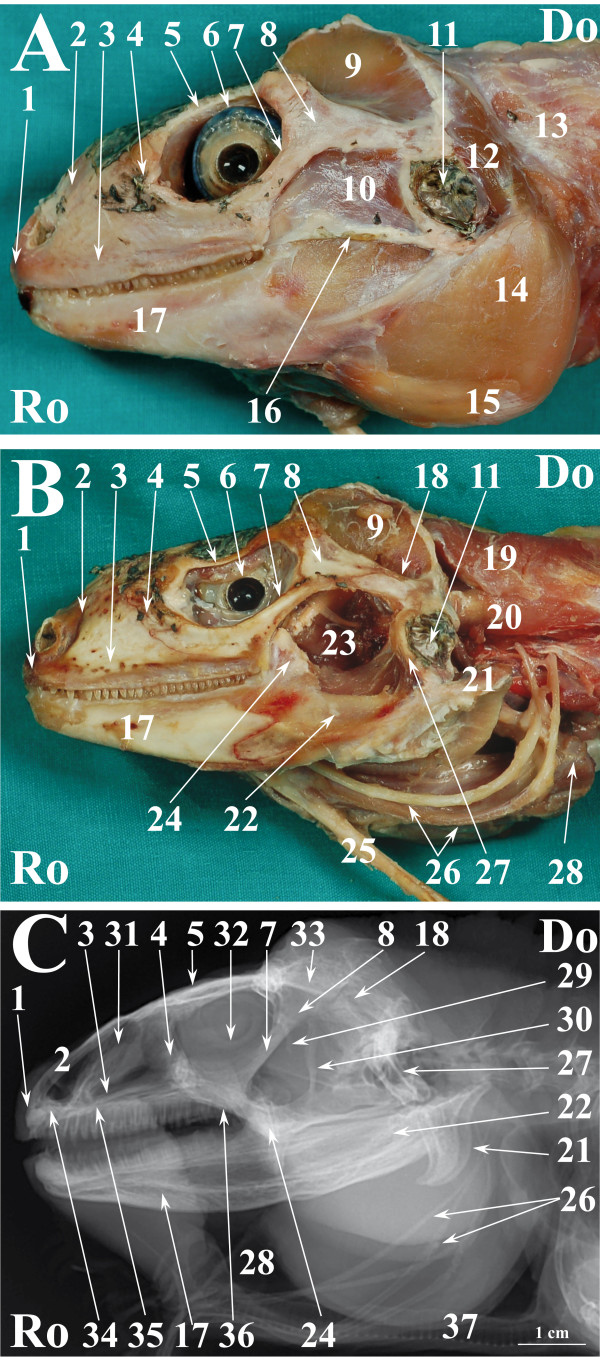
Normal radiographic anatomy of the head of the green iguana in LL projection.A. LL photographic image in a superficial plane of the stratigraphic dissection of the head of an iguana (only skin was removed). B. LL photographic image in a deep plane of the stratigraphic dissection of the head of an iguana. C. LL radiographic projection of the head of an iguana. Ro is rostral, Do is dorsal. 1. Premaxillary bone; 2. Nasal bone; 3. Maxillary bone; 4. Prefrontal bone; 5.Frontal bone; 6. Eye; 7. Jugal bone; 8. Postorbital bone; 9. Musculus adductor mandibulae externus medialis; 10. Musculus adductor mandibulae externus superficialis; 11. Ear; 12. Musculus depressor mandibulae; 13. Musculus episternocleidomastois; 14. Musculus pterygoideus typicus; 15. Musculus intermandibularis posterior; 16. Quadrato-maxillary ligament; 17. Dentary bone; 18. Squamosal bone 19. Musculus trapezius + Musculus clavicle dorsalis; 20. Musculus episternocleidomastoid; 21. Articular bone; 22. Angular bone + Surangular bone; 23. Adductor chamber; 24; Coronoid bone; 25. Ceratobranchial process of hyobranchial skeleton; 26. Hyobranchial skeleton; 27. Quadrate bone; 28. Oesophagus; 29. Pila metoptica; 30. Epipterygoid bone; 31. Nasal glands; 32. Scleral ossicles; 33. Parietal bone; 34. Vomer bone; 35; Palatine bone; 36; Pterygoid bone; 37. Trachea.
